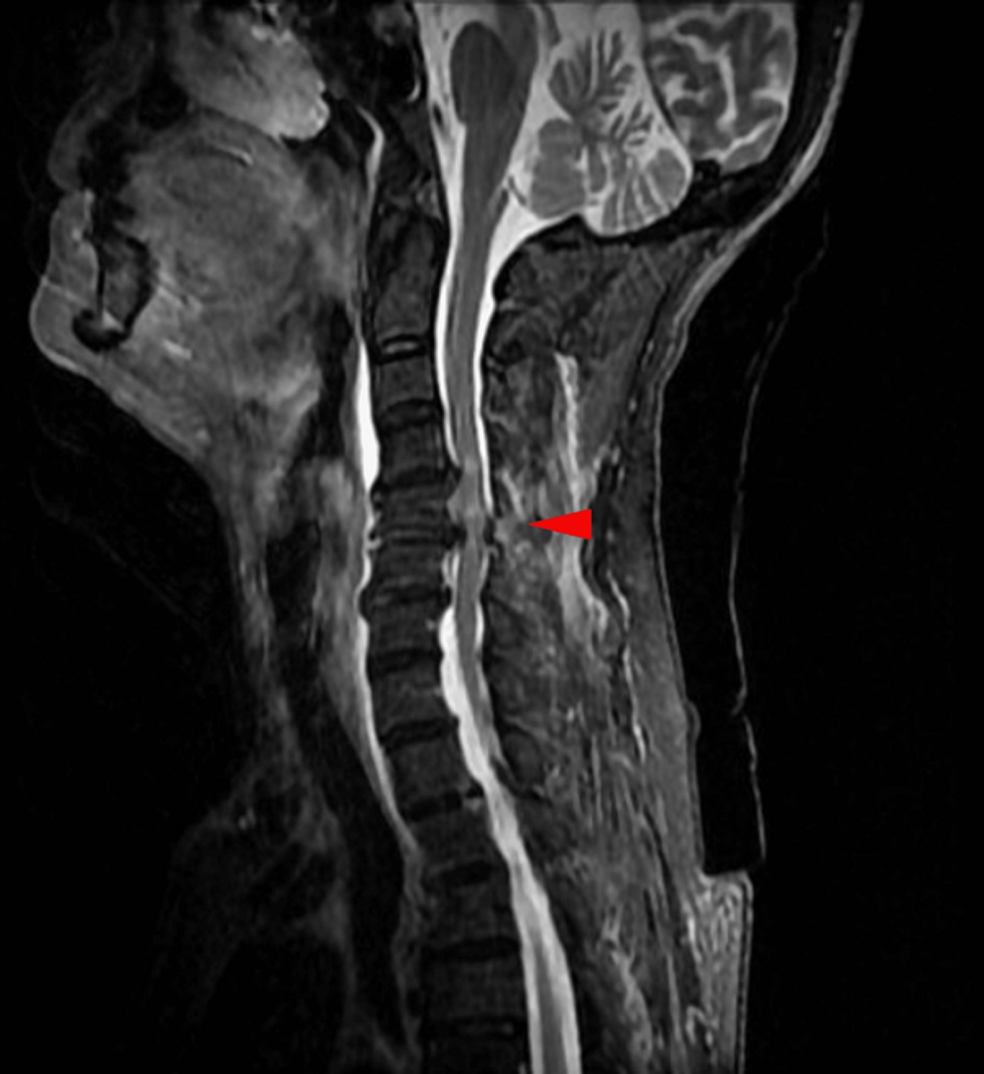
Cervical magnetic resonance imaging The image shows spinal cord injury and spinal stenosis at C4/C5 and C5/C6 (arrowhead).
